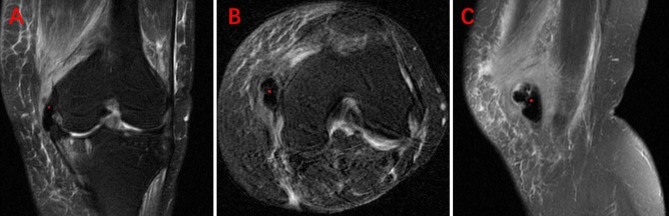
Preoperative MRI of the left knee. (A) Coronal T2 weighted image (T2WI); (B) transverse axis proton density weighted image (PDWI); (C) sagittal T2WI. *Pellegrini–Stieda lesion.
